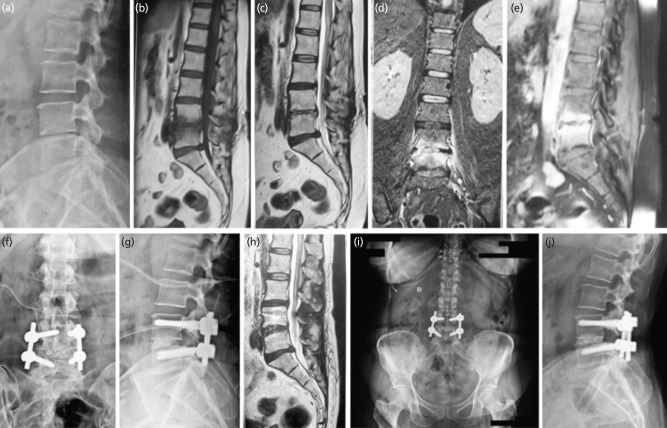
Female 48 years, Operated micro lumbar discectomy for Lumbar Disc herniation L4-5. (a) Lateral radiograph before TLIF surgery. Irregularity of endplate and disc space reduction noted. (b-d) MRI T1-T2 sagittal, coronal sequence showing intra-discal abscess, vertebral body edema, with end-plate destruction. (e) Contrast MRI showing soft tissue, body and disc enhancement. (f, g) Antero-posterior and lateral radiograph showing transforaminal lumbar inter body fusion executed with only interbody bone graft and pedicle screws. (h) MRI T2 sagittal at 3 months follow-up showing no active signs of infection. (i, j) 80 months final follow-up antero-posterior and lateral radiographs showing stable union and reconstruction.
